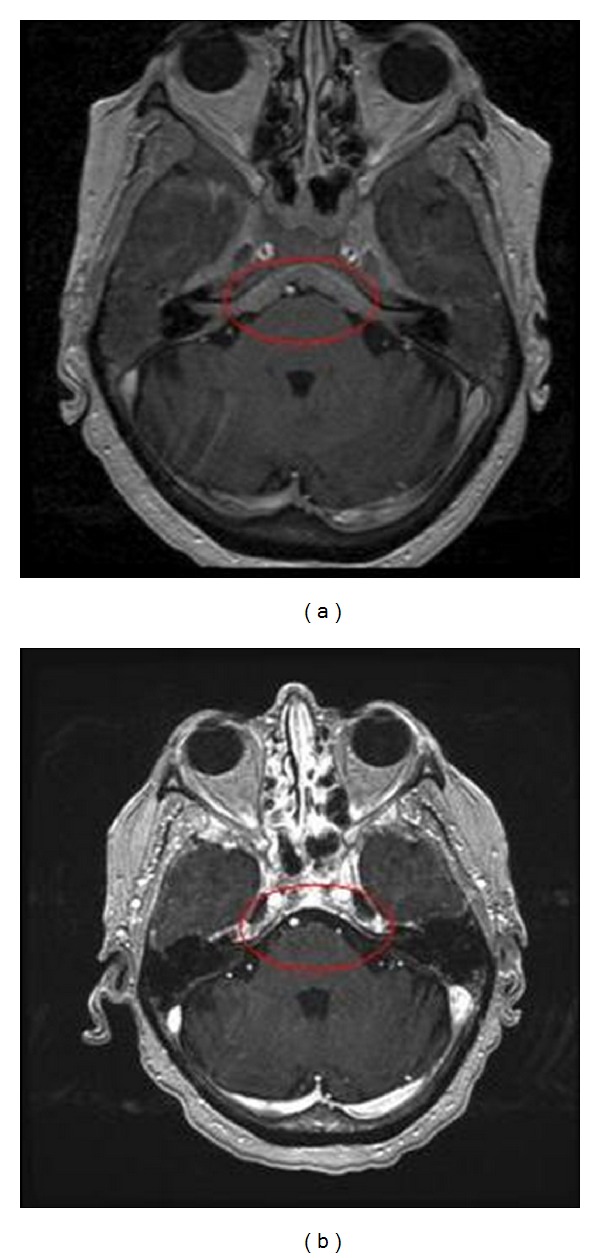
Magnetic resonance imaging of the brain demonstrating thickening of the skull base (a) that has responded to therapy and appears normal in subsequent imaging (b).
